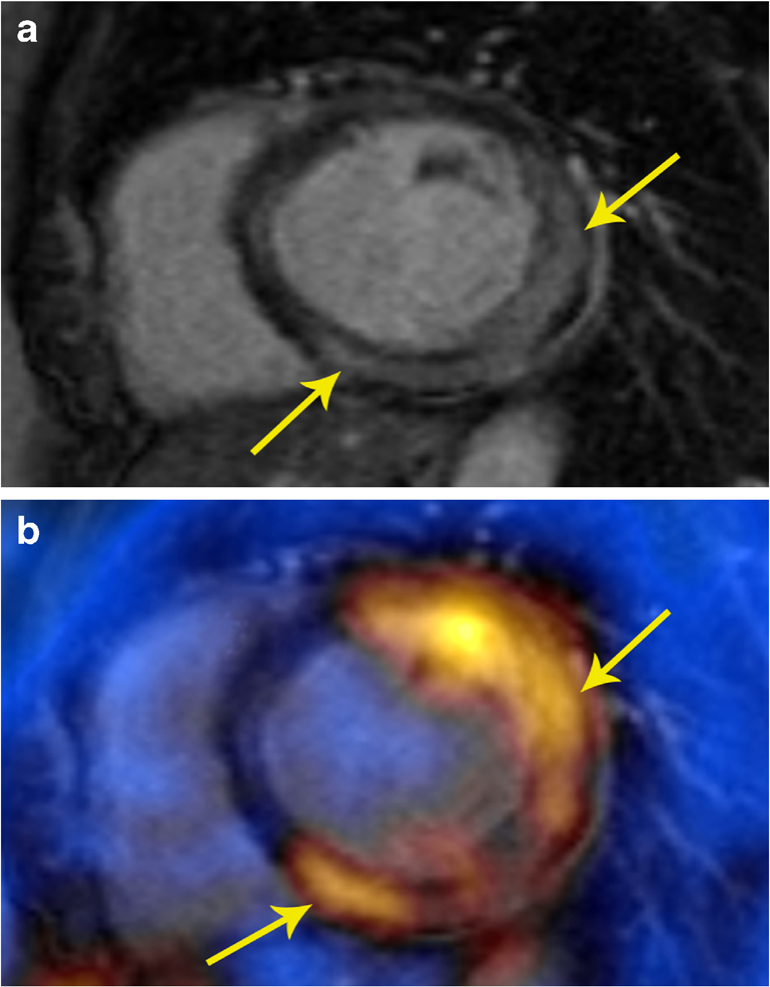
Myocarditis. a Short-axis delayed enhancement MRI in a patient with acute onset chest pain and elevated enzymes shows mid-myocardial enhancement in the mid lateral and inferior segments (arrow). b Fused PET/MR image obtained after glucose diet shows patchy areas of intense uptake in the lateral and inferior wall, indicative of active inflammation
